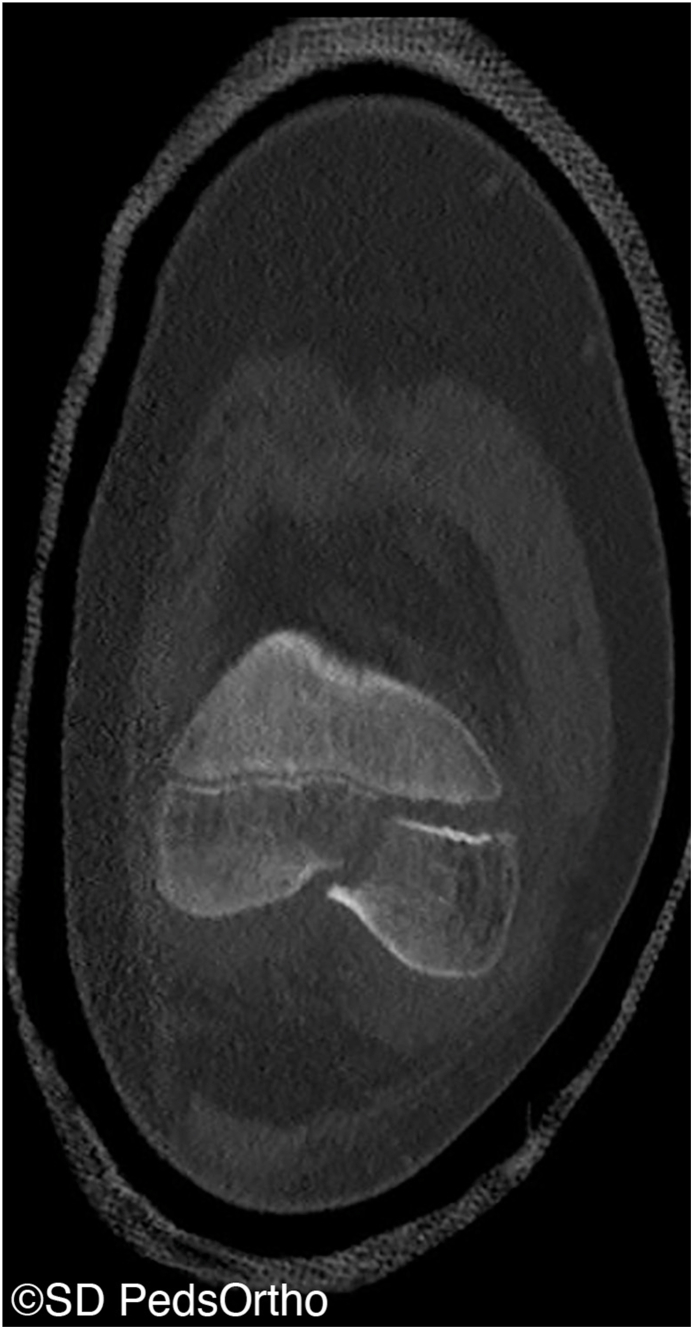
Coronal view of the distal femur Salter-Harris type III fracture from CT imaging. *CT, computed tomography.*
